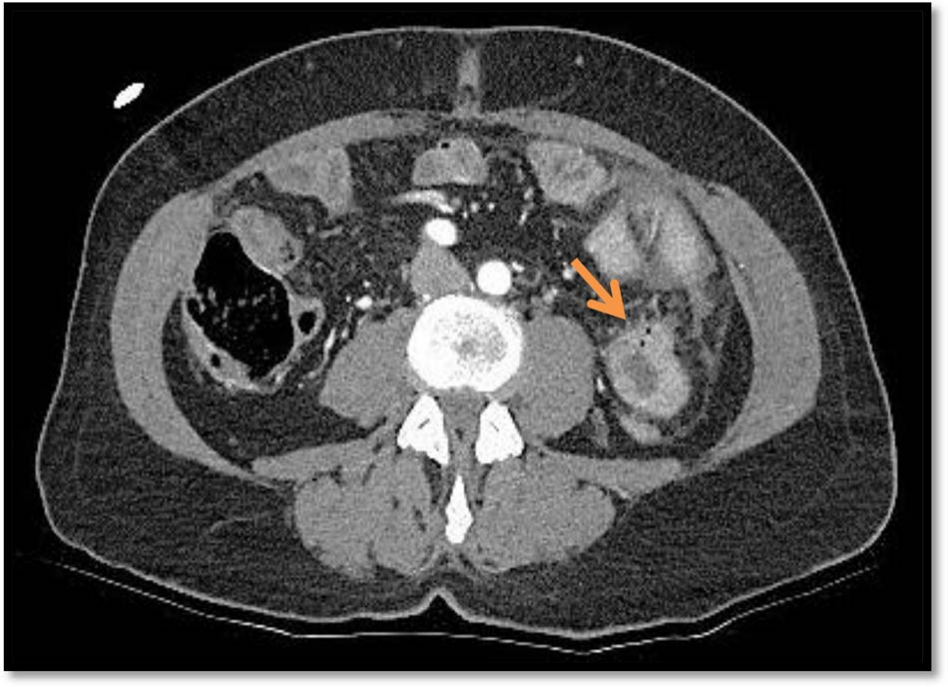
Free air bubbles on the CT scan in the emergency department.
